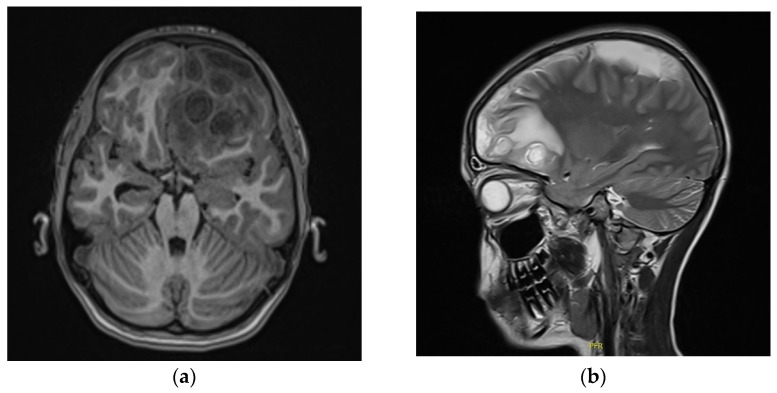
Magnetic resonance imaging (MRI) of the head on 28 December 2021 revealed: (a,b) significant progression of brain abscesses with edema and mass effect leading to displacement of midline structures at the right side.
